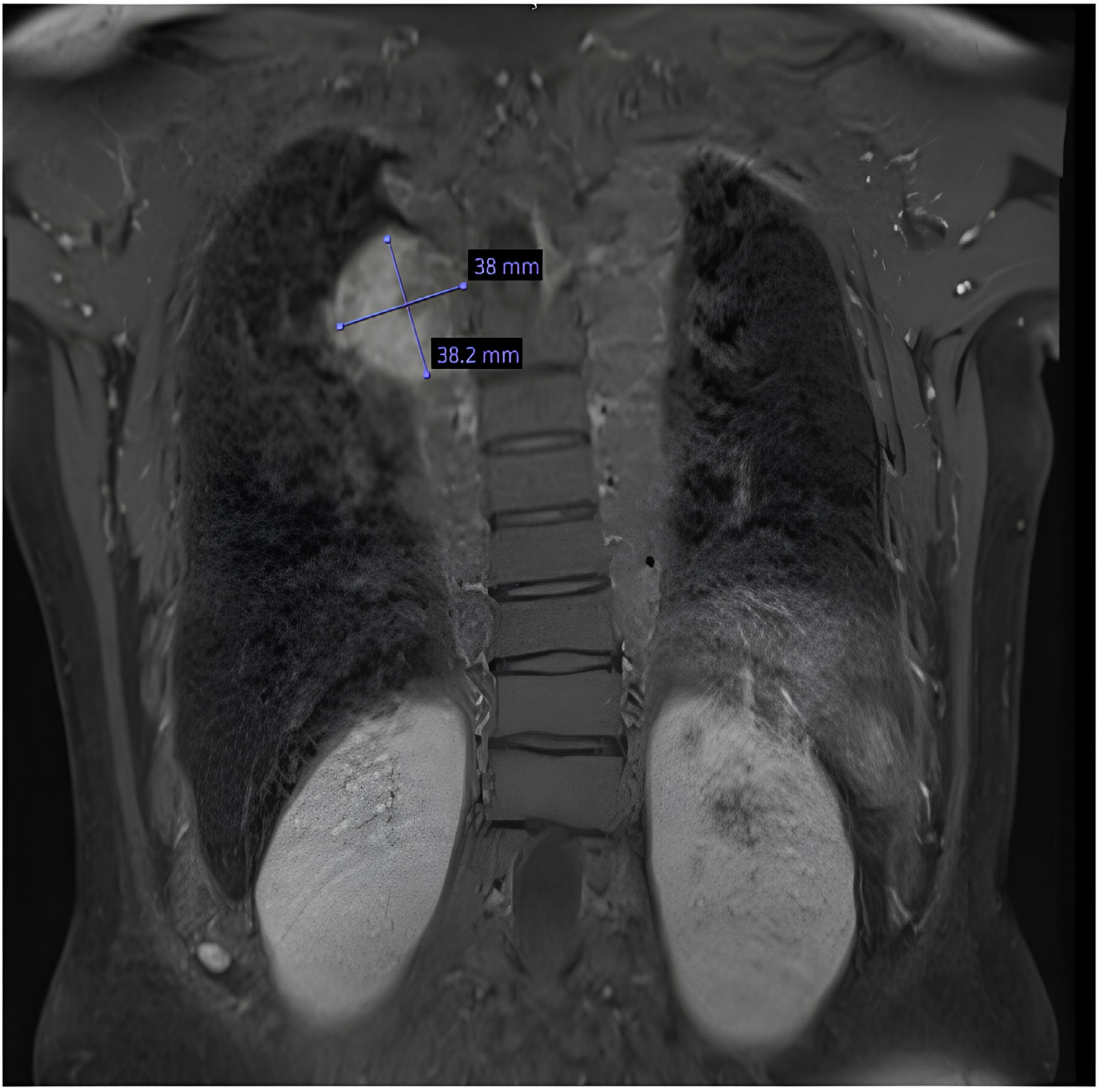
MRI thoracic spine dated October\2022.
